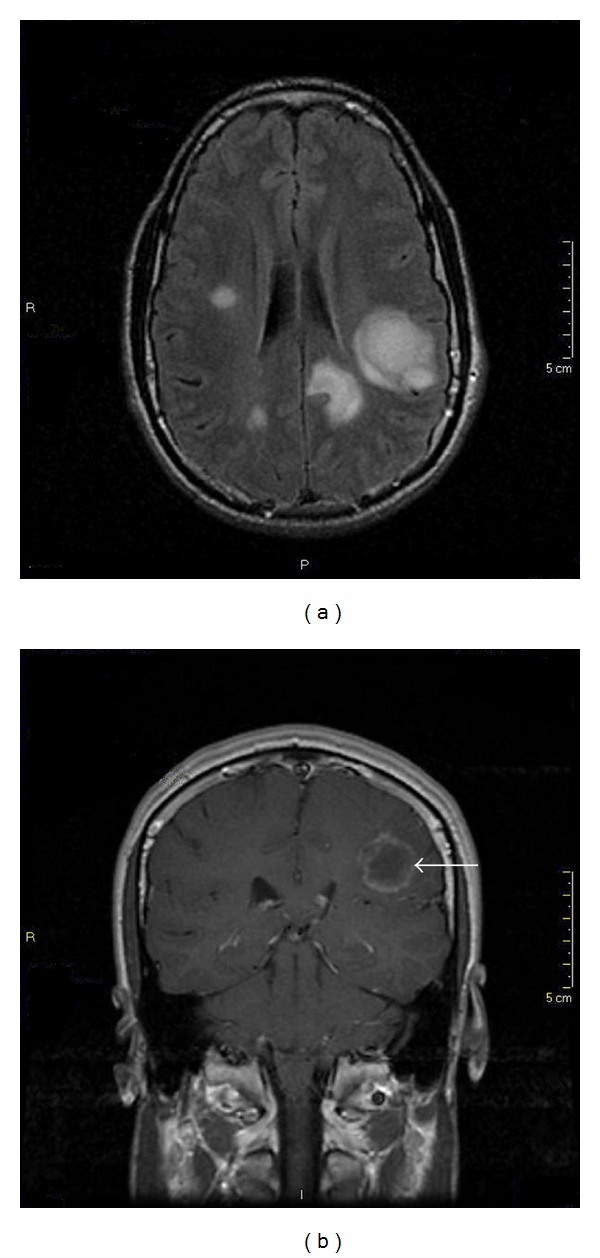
Initial MRI scan of the brain. An axial FLAIR image shows multiple hyperintense bilateral brain masses involving mainly left parietal lobe and splenium of corpus callosum (a). A coronal T1-weighted image (b) obtained after contrast administration shows gadolinium rim-enhancement of the main lesion (arrow).
